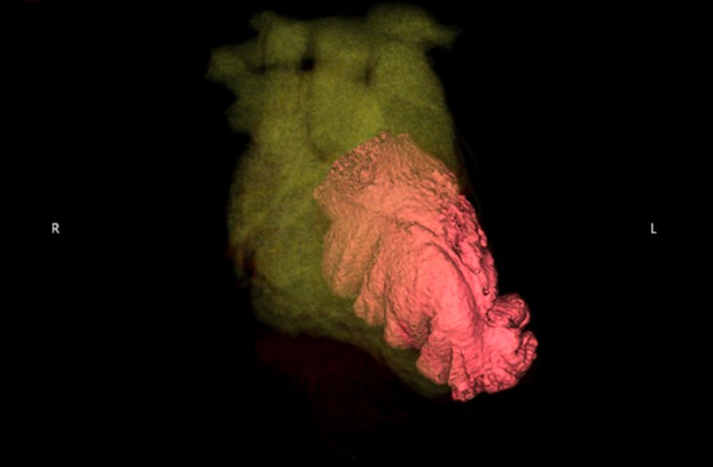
3-Dimensional Images Created From Computed Tomography Angiography The left ventricular external structure is highlighted in red.
